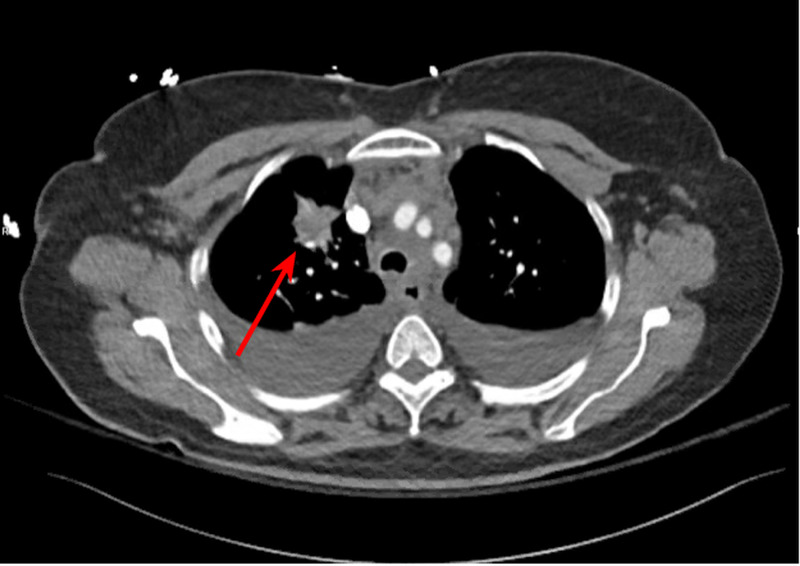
CT angiogram of the chest showing a spiculated mass in the right upper lobe (arrow).
